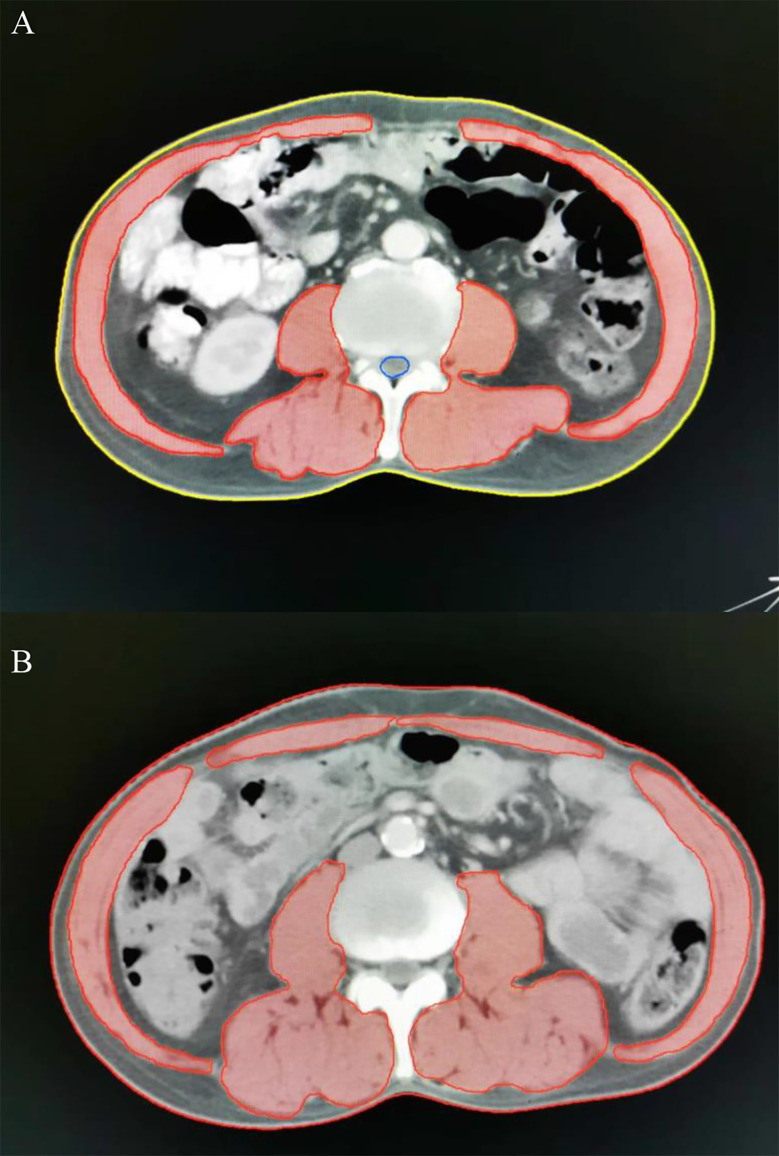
Axial computed tomography (CT) images at the level of L3 with skeletal muscle highlighted in red. Representative CT images in patients with (A, a-67-year-old male patient, SMA =122.85 cm^2^, BMI = 21.38 kg/m^2^, SMI=41.04 cm^2^/m^2^) and without sarcopenia (B, a-62-year-old male patient, SMA =158.64 cm^2^, BMI = 21.51 kg/m^2^, SMI=56.88 cm^2^/m^2^). SMA, skeletal muscle area; BMI, body mass index; SMI, skeletal muscle index.
